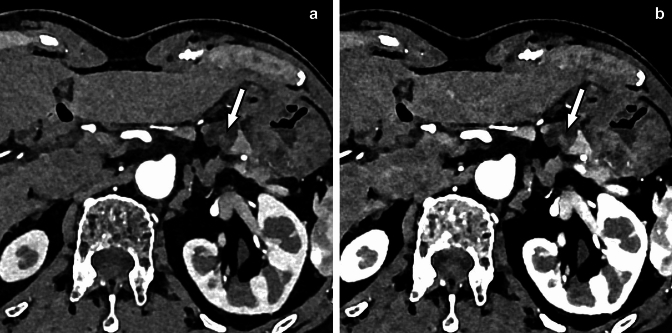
A cystic lesion with a mural nodule (arrows) is seen in the tail of the pancreas on the pancreatic parenchymal phase (arterial phase) photon-counting CT images at 70 (A) and 50 keV (B). The cystic lesion is consistent with an intraductal papillary mucinous neoplasm. The 50 keV image (B) shows the enhancement of the mural nodule more clearly than the 70 keV image (A). Such a nodule is a sign of malignancy *(i.e.*, high-risk stigmata)
